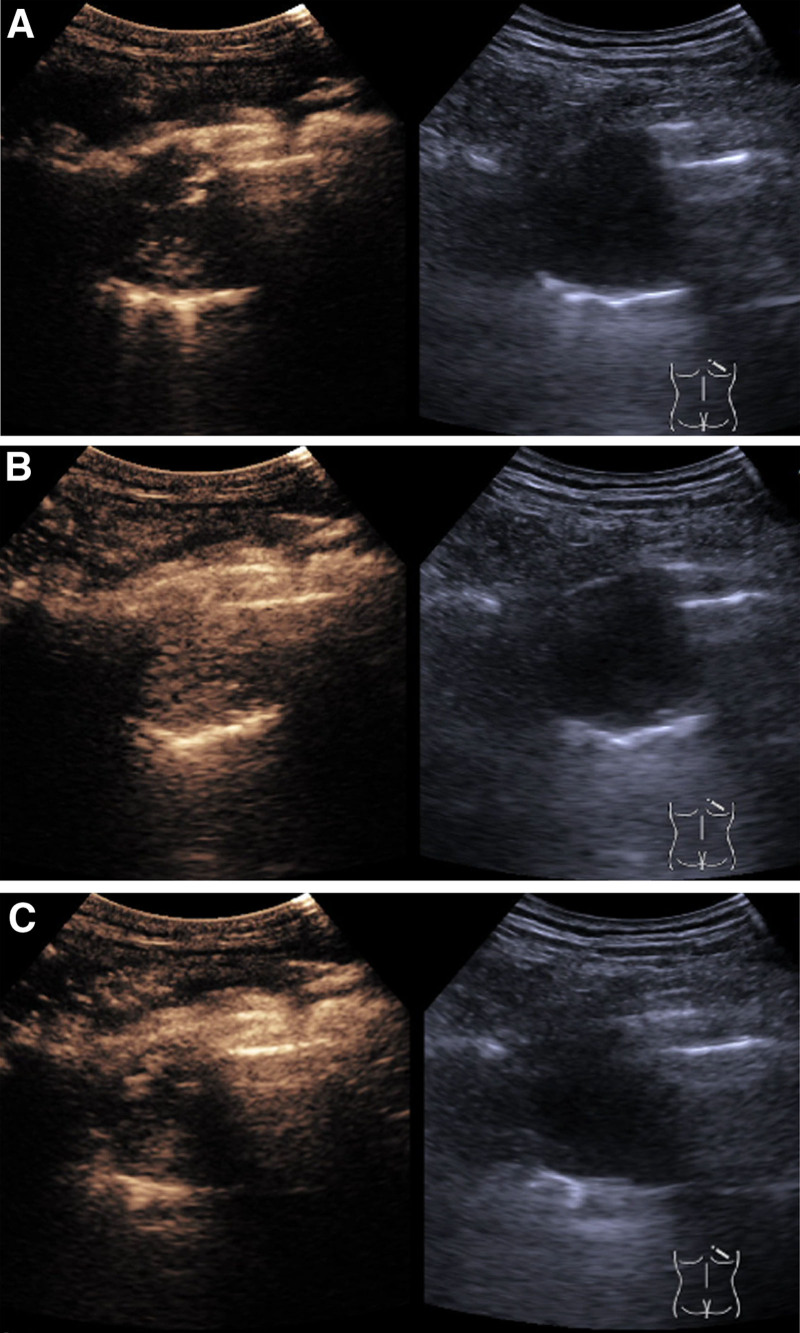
(A) Ultrasound contrast enhanced at 14 seconds, with thick and twisted vascular branches around the mass. (B) At 27 seconds, the enhancement reached its peak, with most of the masses showing high enhancement and some areas without enhancement visible inside. (C) At 1 minute and 12 seconds, the contrast agent gradually decreased and the mass showed uneven low enhancement.
